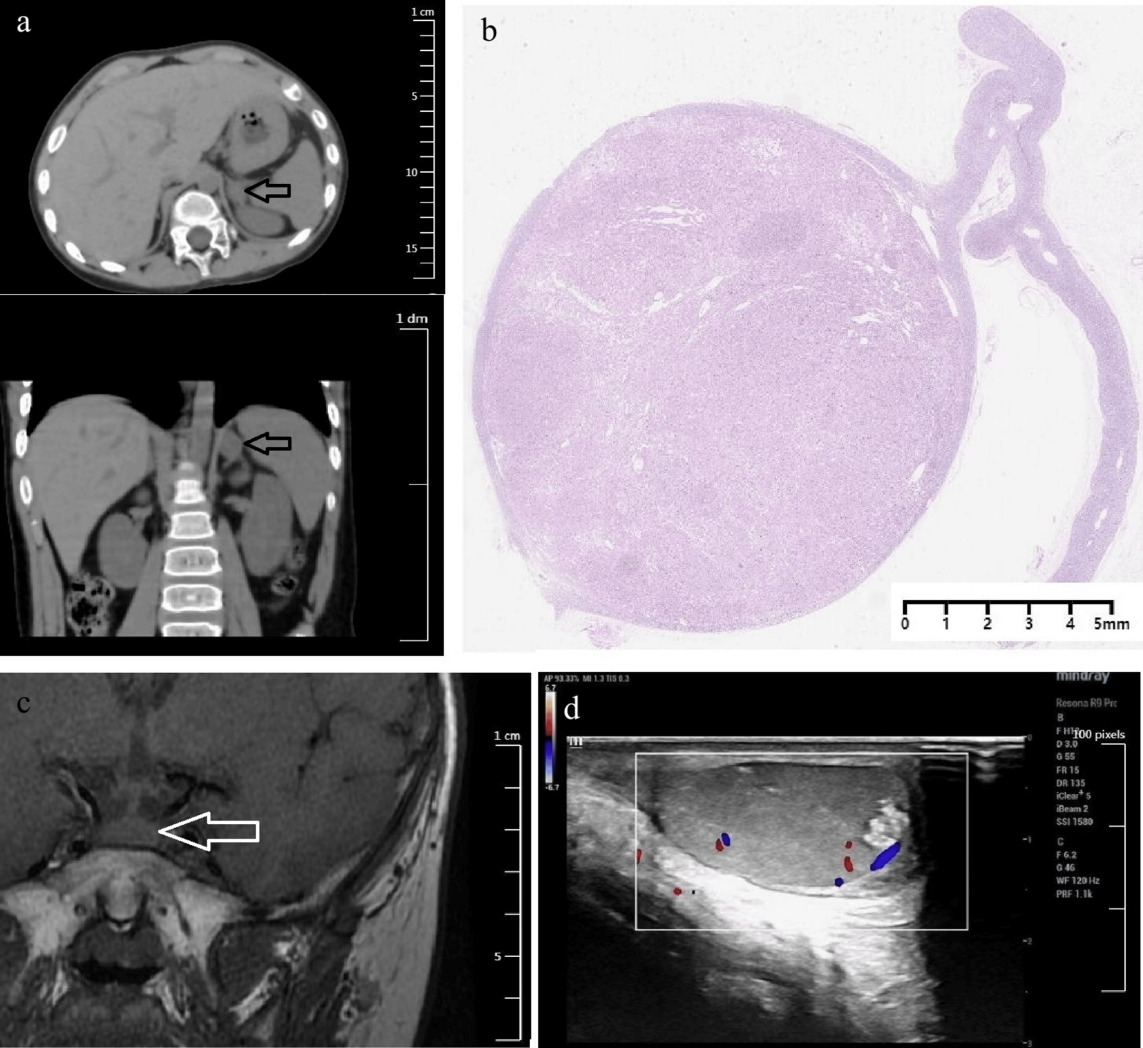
Representative imaging and pathologic findings of multifocal lesions in the adrenal gland, pituitary, and testis (a) Adrenal CT scan images. (b) Pathology of the left adrenal. Immunological parameters: CK (-), EMA (-), Vim (-), CgA (-), SyN (+), CD56 (-), S-100 (-), Ki67 (+) 3%. (c) Pituitary MR image. (d) Testis ultrasound image.
